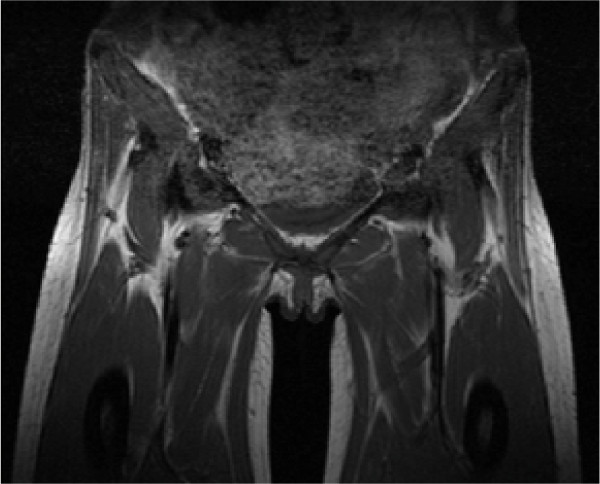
Coronal T1-weighted MRI of the pelvis and hips - repeat imaging.
